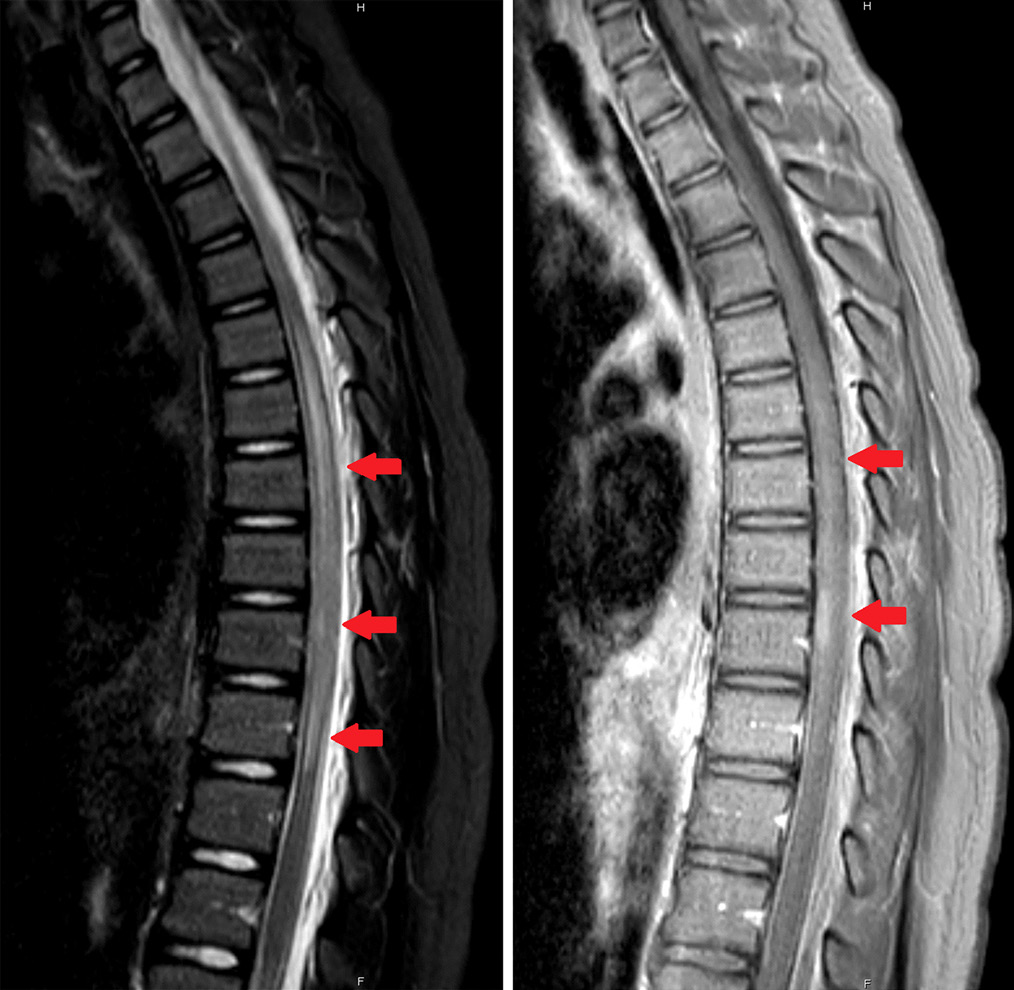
Initial thoracic spine MRI findings. Left: Sagittal STIR image demonstrating multiple longitudinally extensive spinal cord lesions (red arrows). Right: Sagittal T1 post-contrast image demonstrating corresponding lesion enhancement. STIR, short tau inversion recovery.
